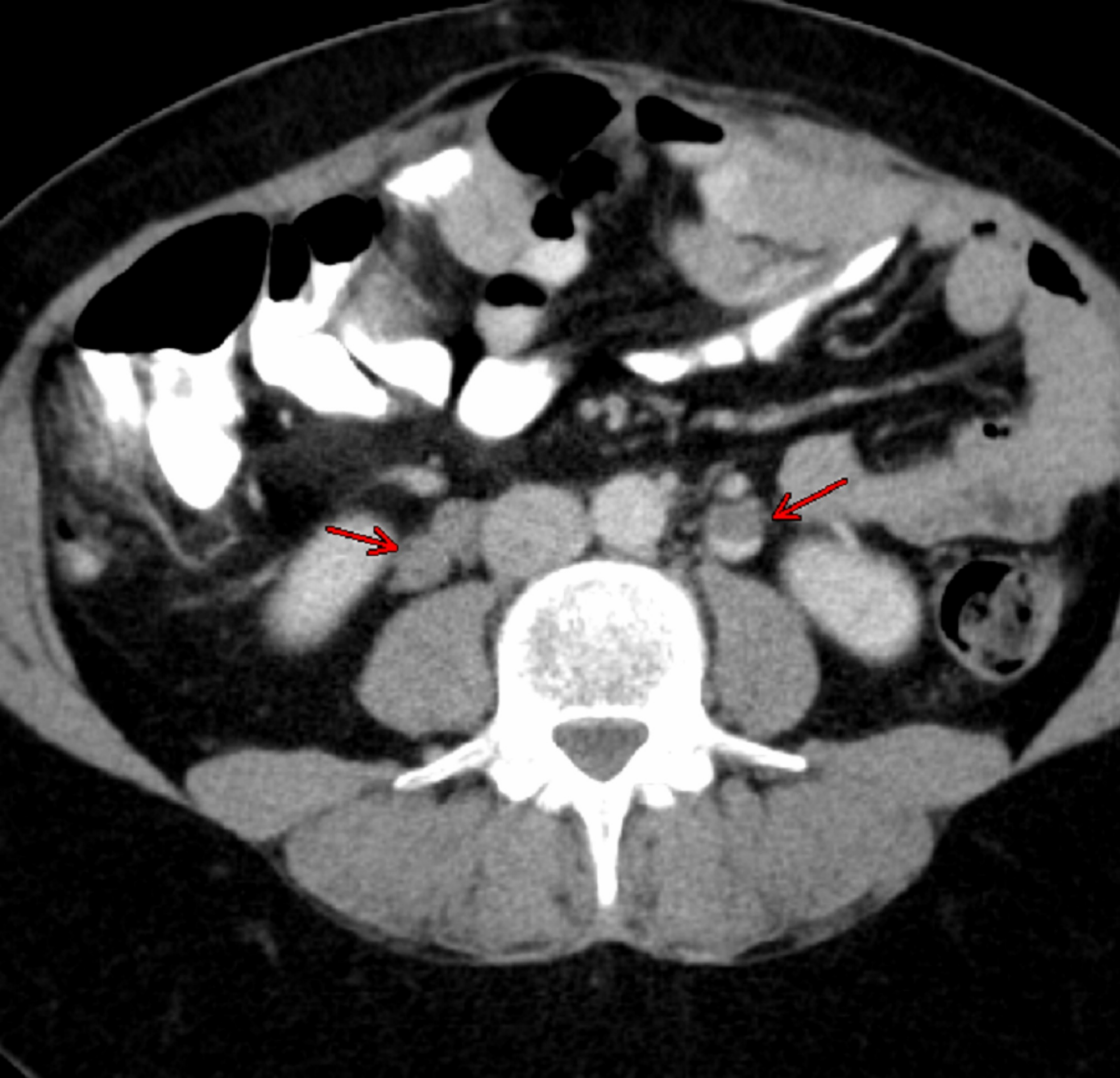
CT image showing filling defect in the right and left gonadal vein (red arrows) CT: computed tomography
